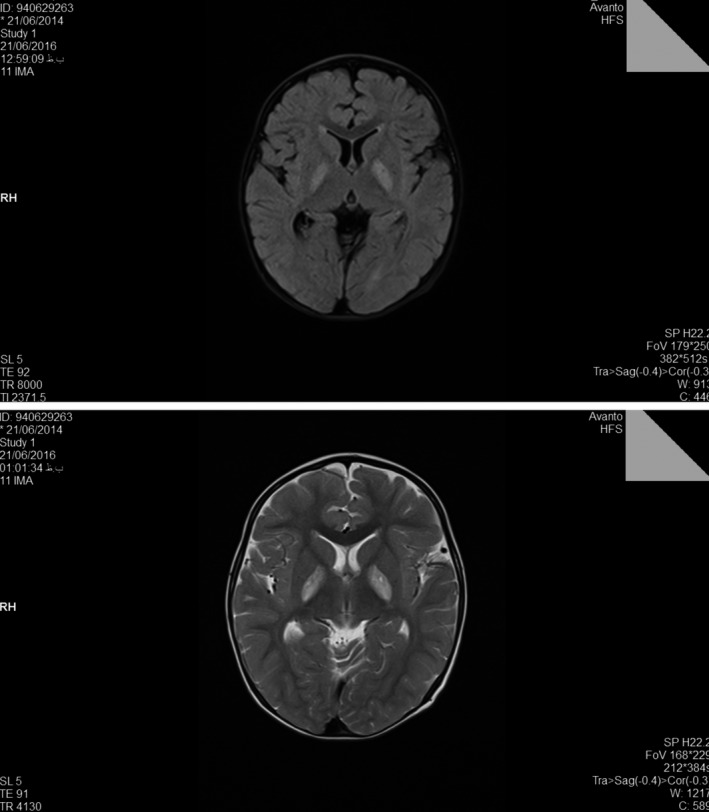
Brain MRI at age 2 y showed bilateral high signal lesions in the globus pallidus
